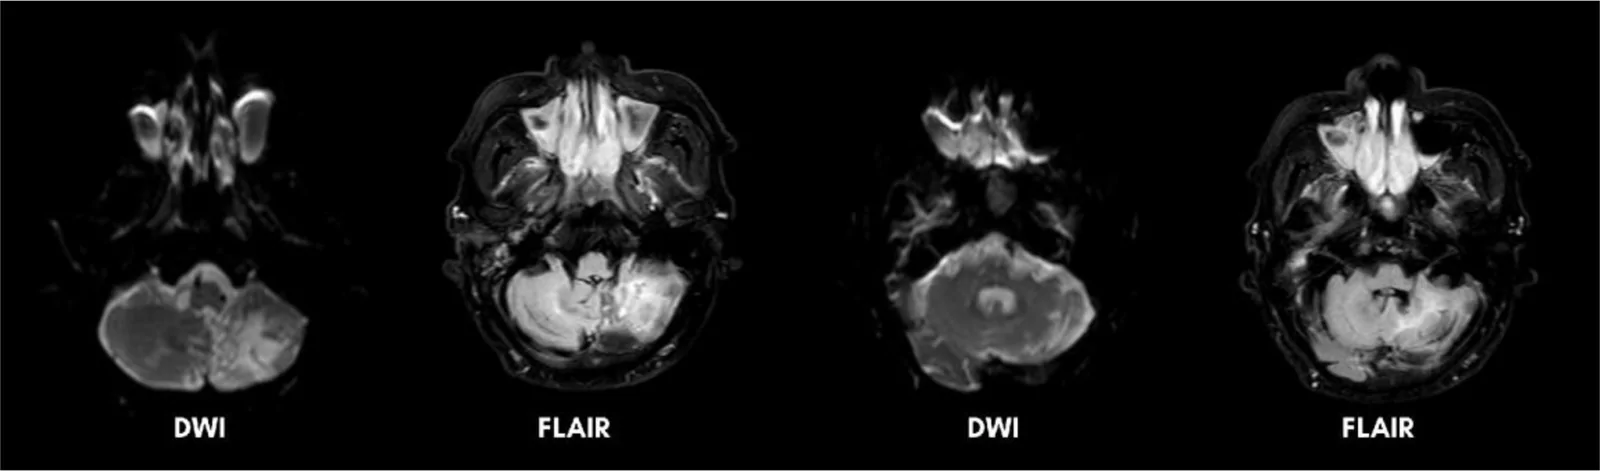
Follow-up non-contrast enhanced brain magnetic resonance imaging (MRI) at 1.5 years demonstrating no diffusion restriction on diffusion-weighted imaging (DWI), with a predominantly hypointense lesion and a hyperintense rim on T2-weighted fluid-attenuated inversion recovery (T2W FLAIR), consistent with a chronic cerebellar infarction, predominantly involving the left cerebellar hemisphere.
